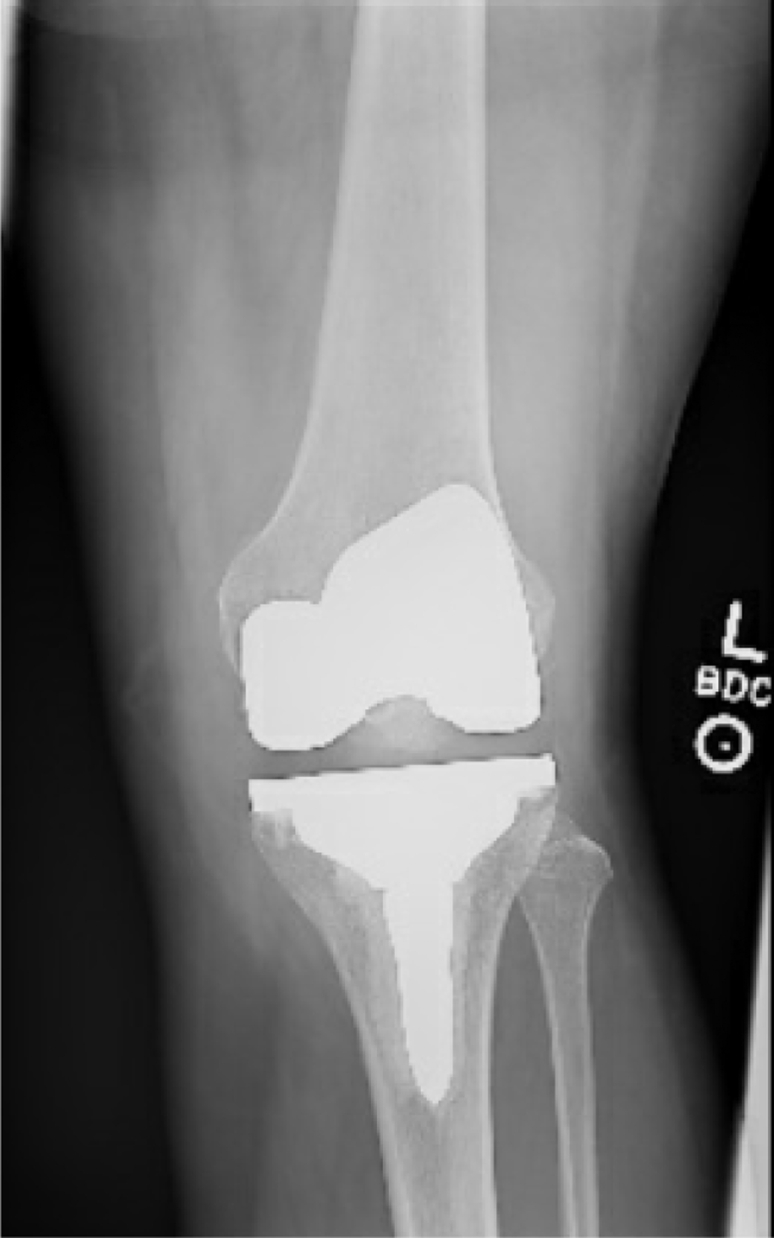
X-ray image showing resolved swelling in the medial portion of the knee 4 months after UBM application.
